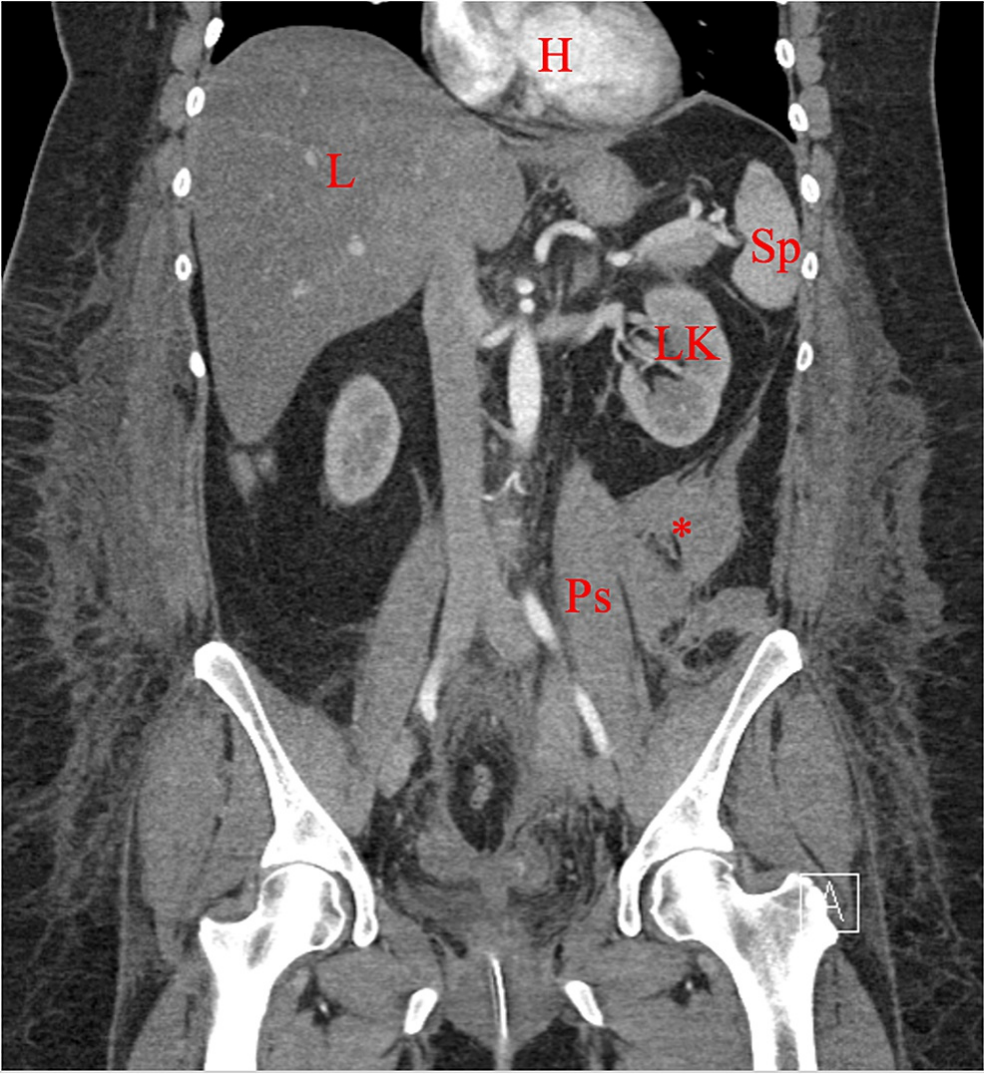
Coronal CT demonstrating retroperitoneal hematoma at the level of the femoroacetabular joint. H, heart;L, liver; Sp, spleen; LK, left kidney; *, retroperitoneal hematoma; Ps, psoas muscle
